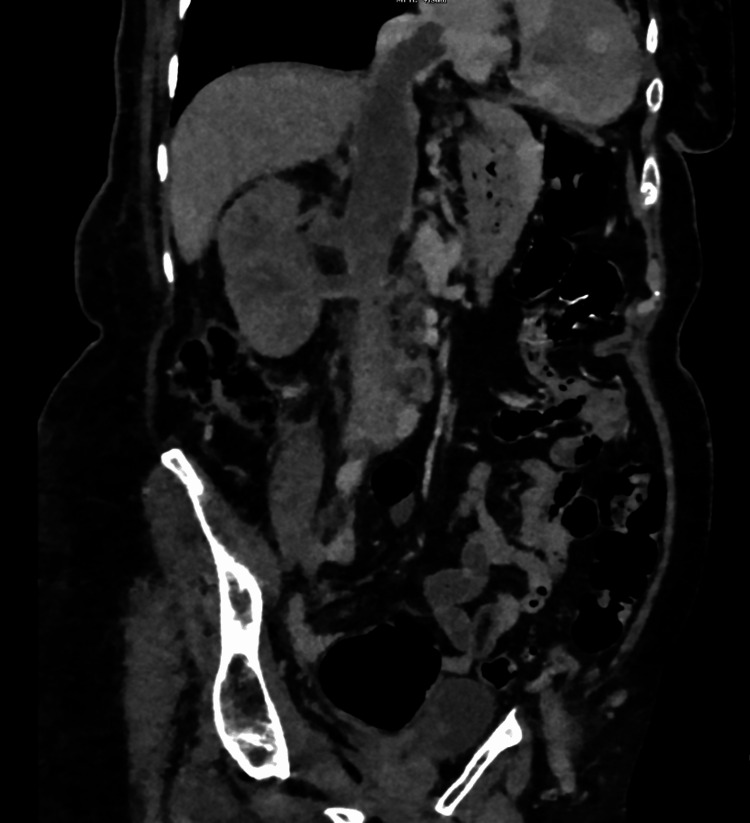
Coronal CT abdomen and pelvis CT demonstrating tumour thrombus extending up the inferior vena cava.
